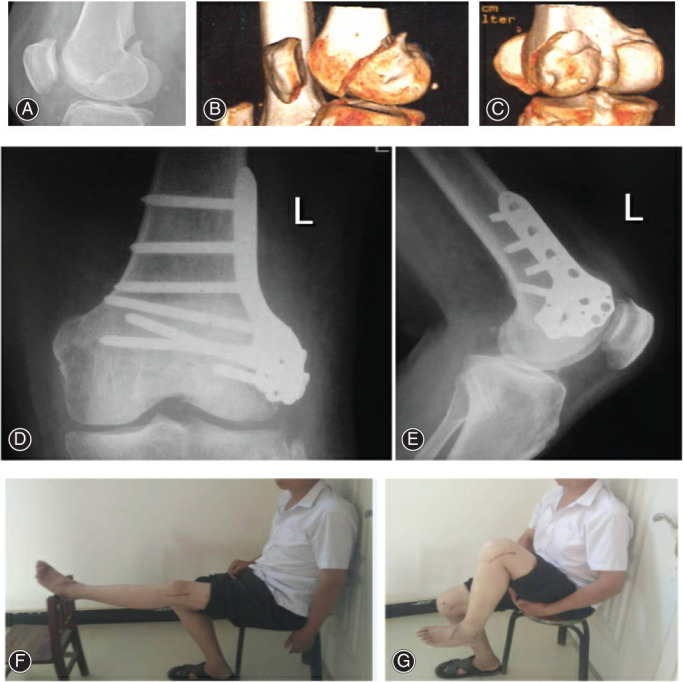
A 52‐year‐old male patient sustained a left Hoffa fracture in a motor vehicle accident. Lateral radiograph and CT of left knee showed a type Letenneur III lateral Hoffa fracture (A–C). Radiographs showed that the patient underwent open reduction and internal fixation, and a DFCLP alone was placed at the lateral distal femoral condyle (D, E). Twenty‐four months after the operation, nearly 0°–120° range of motion was obtained in the patient with satisfactory functional results (F, G)
